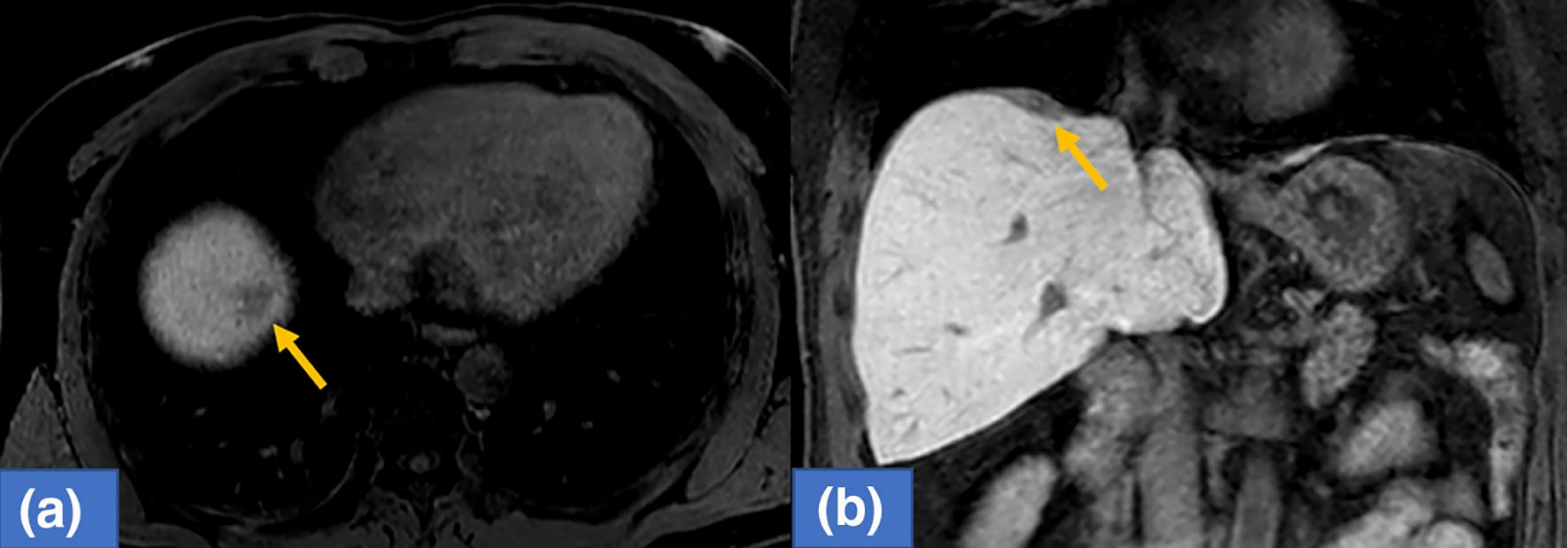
EOB-MRI revealed a hypointensity tumor located just below the diaphragm at liver segment 8 on hepatobiliary phase (arrow). (a: axial sections, b: coronal sections)
